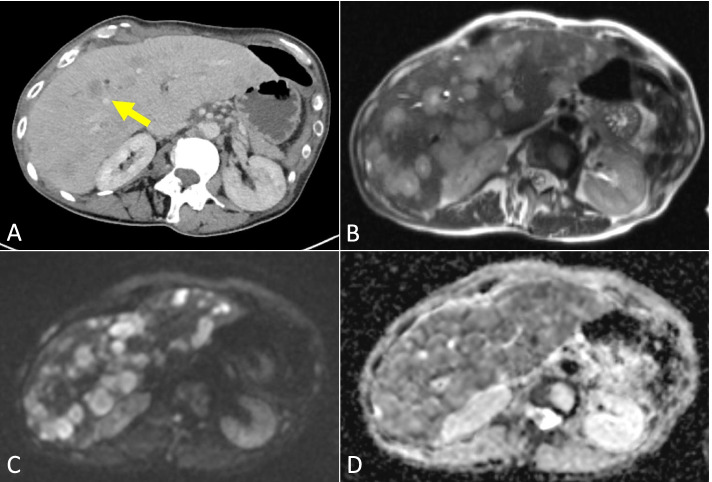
Disease underestimated by CT. 55-year-old female with ER- PR- positive HER-2 negative infiltrating ductal carcinoma with axillary nodal and liver metastases. CT demonstrates bi-lobar liver metastases but significantly underestimates the burden of disease when compared to the WBMRI performed 2 days later. CT (A) demonstrates liver metastases measuring up to 18 mm within segment VIII (yellow arrow) however axial T2 (B), high b-value (b900) DWI sequences (C) and corresponding ADC map (D) from the WBMRI study demonstrates many more lesions not appreciated on CT
